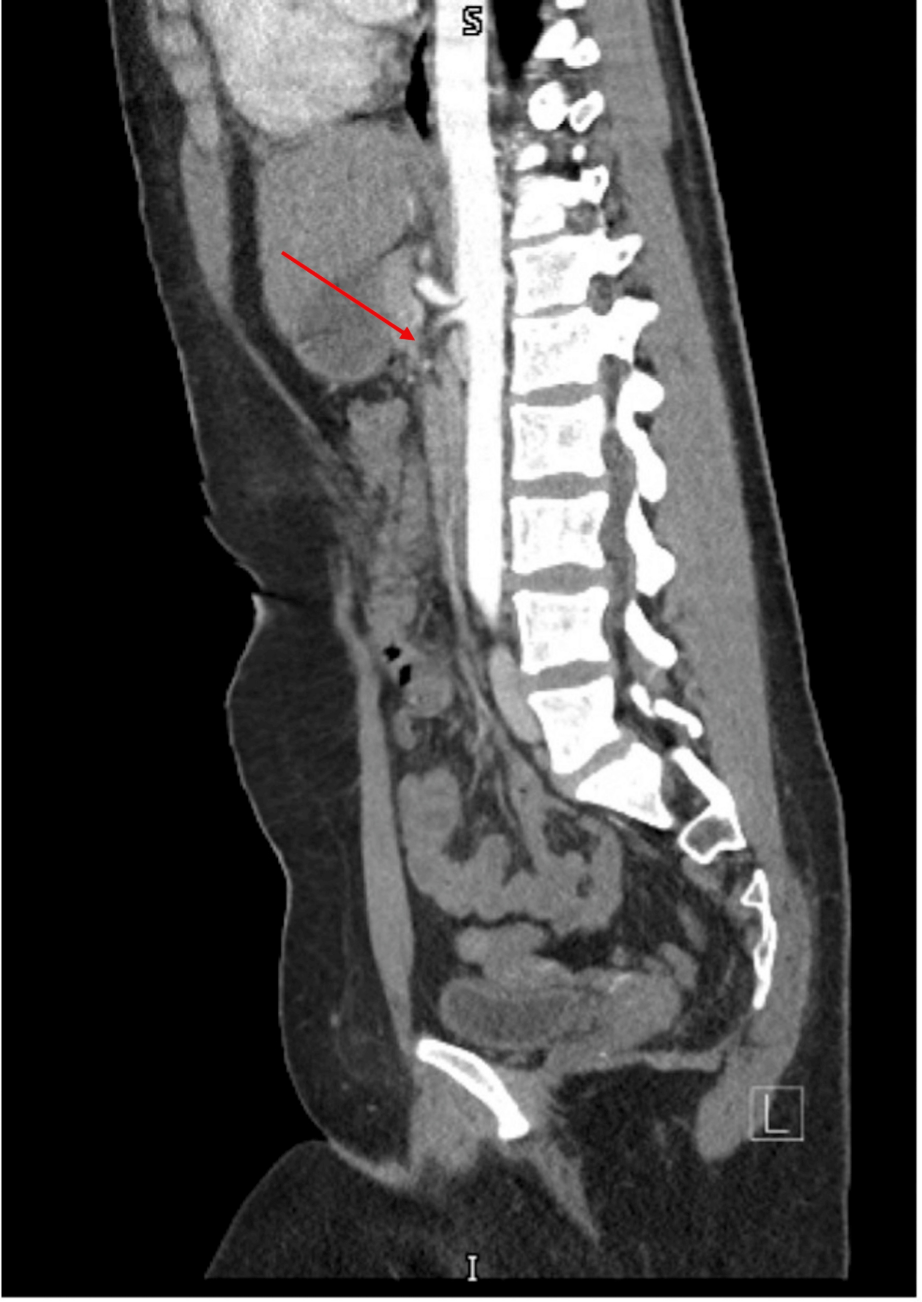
CT angiographic findings in median arcuate ligament syndrome (MALS) Sagittal view of a CT angiogram of the abdomen demonstrating the characteristic morphology of the celiac trunk in MALS. The superior compression of the celiac artery by the MAL results in narrowing and a hooked appearance of the vessel.
